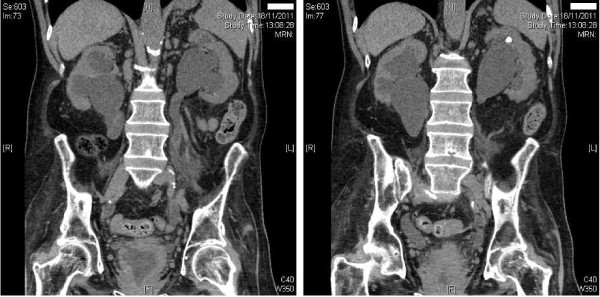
A; (left panel) Computed tomography (coronal view) confirmed moderately severe bilateral hydronephrosis and hydroureter. B (Right panel) Computed tomography (coronal view) revealed moderately severe bilateral hydronephrosis. A small calculus was present in the upper pole calyx of left kidney.
